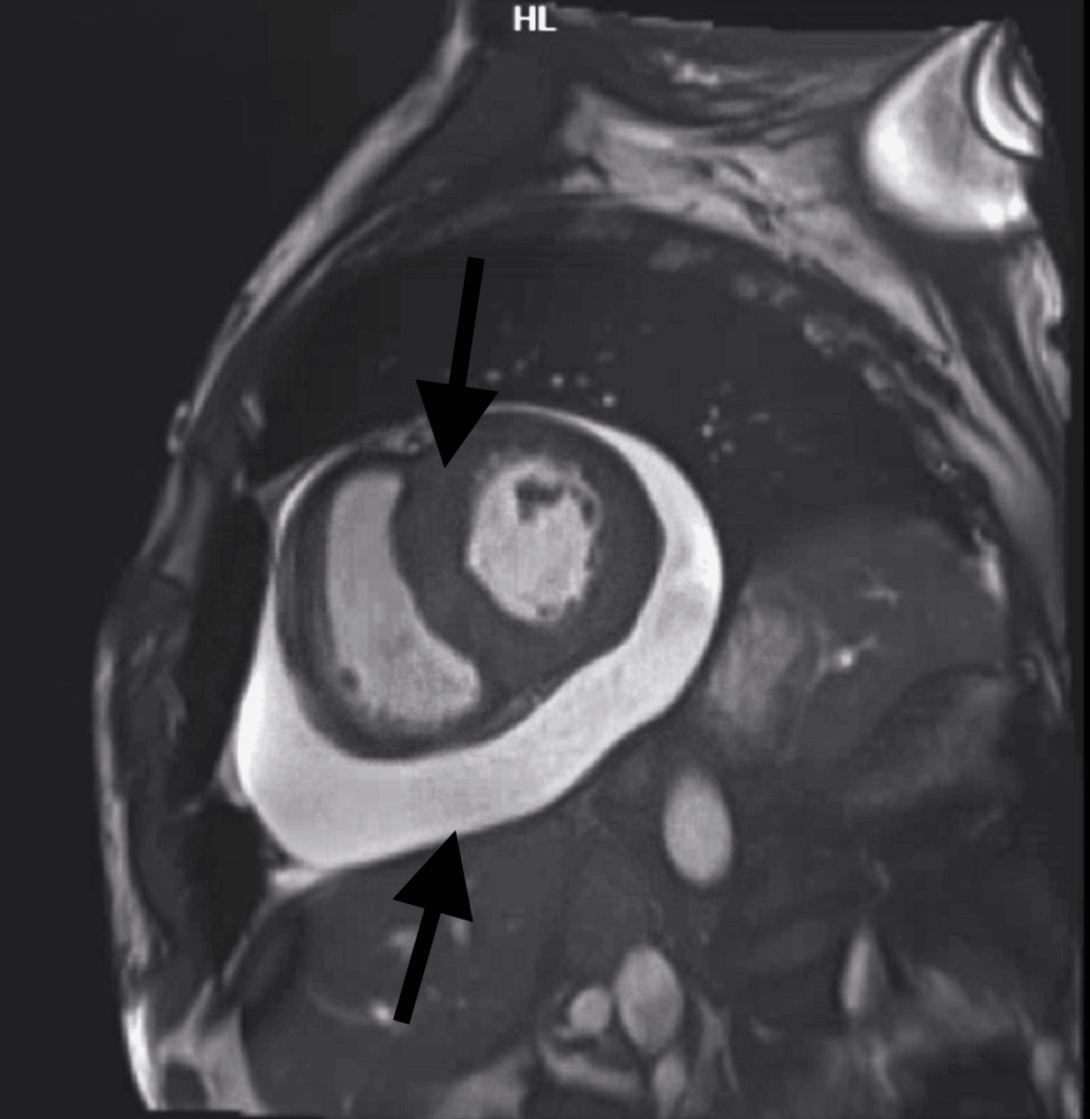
cMRI showing severe LVH and pericardial effusion (black arrows) cMRI: cardiac magnetic resonance imaging; LVH: left ventricular hypertrophy
